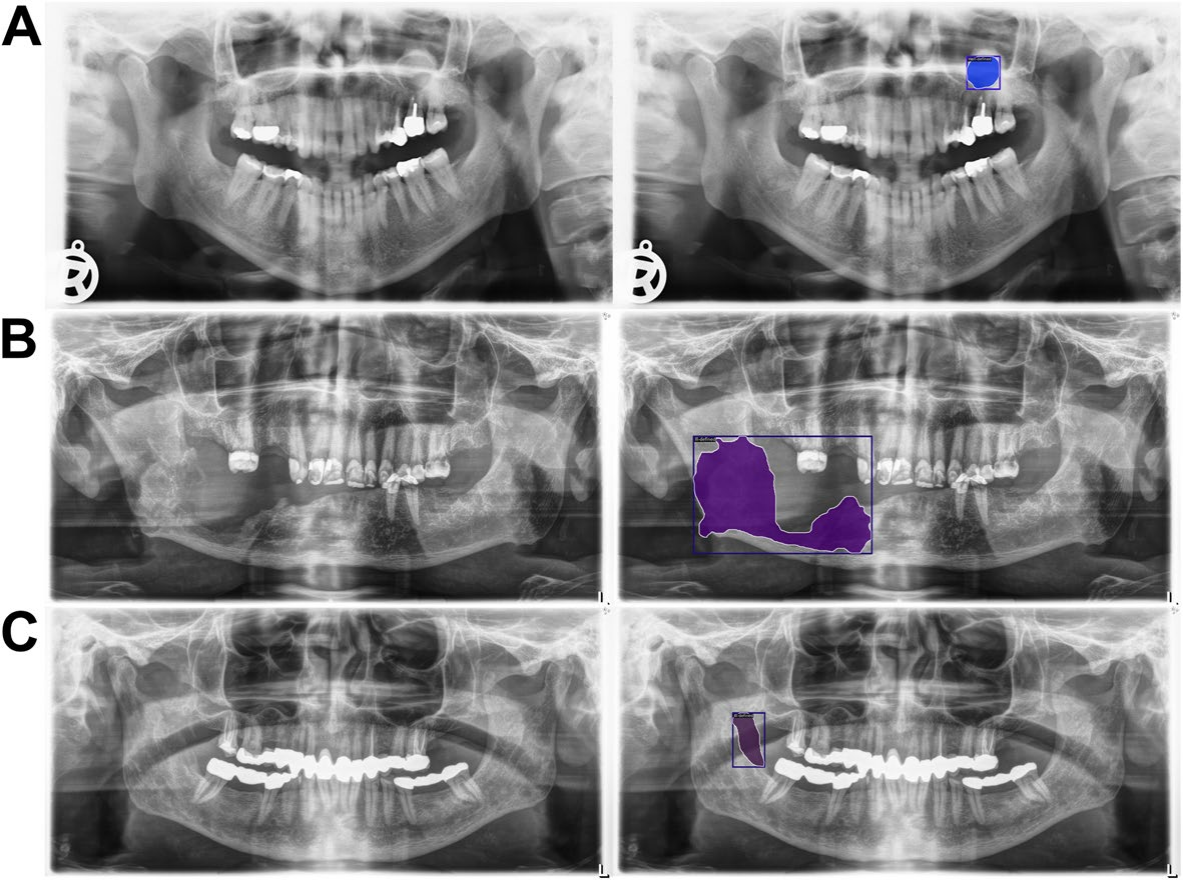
Examples of False-negative predictions. The panoramic radiographs (PRs) in the left column show cases where the most effective model—Mask R-CNN with a Swin-Tiny backbone—missed osteolytic lesions, which are displayed in the right column. In image A, the model missed a lesion in the upper jaw near the maxillary sinus. Image B shows a large osteolytic lesion in the mandibular angle that the model missed. In image C, a radiolucent band caused the model to miss the underlying lesion. These examples highlight common sources of false negatives, including radiographic artifacts and the maxillary sinus
